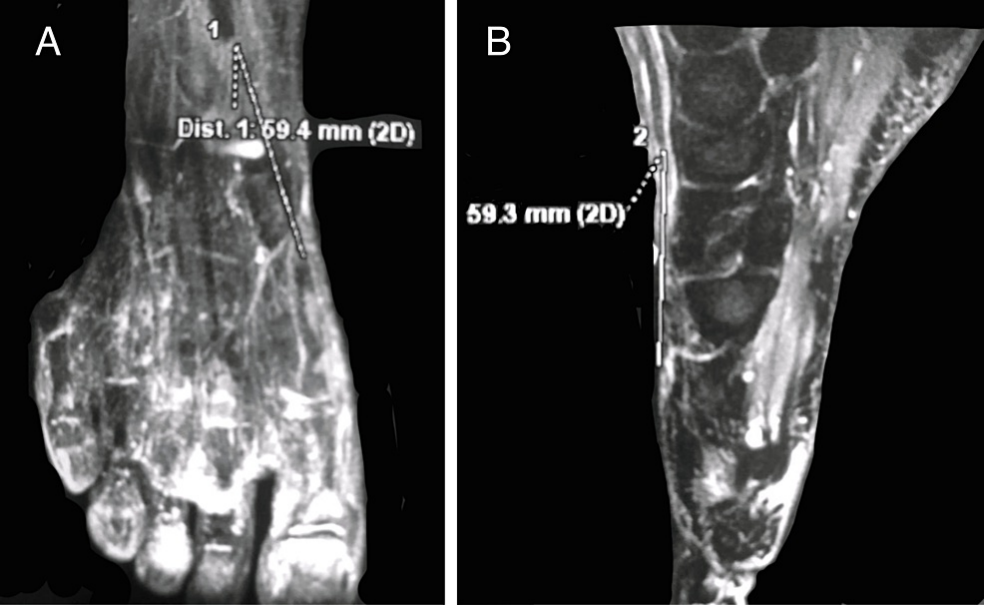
MRI images (A: coronal view; B: sagittal view) showing a 5.9 cm gap between the two ends of the ruptured extensor hallucis longus (EHL) tendon
